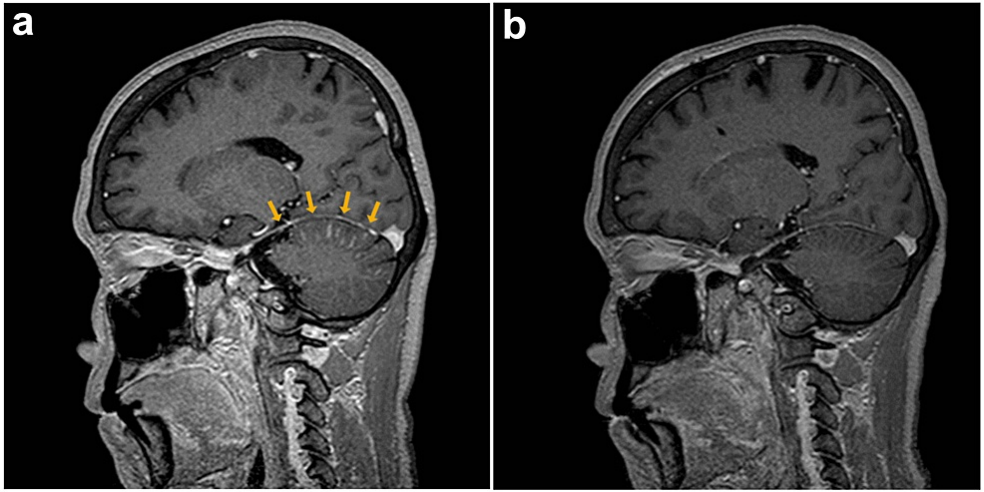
Contrast-enhanced MRI of the brain (a) Contrast-enhanced MRI of the brain at the diagnosis of leptomeningeal metastasis shows leptomeningeal enhancement around the cerebellum (yellow arrows). (b) Contrast-enhanced MRI one month after the initiation of intrathecal trastuzumab shows a decrease in enhancement around the cerebellum. MRI; magnetic resonance imaging
